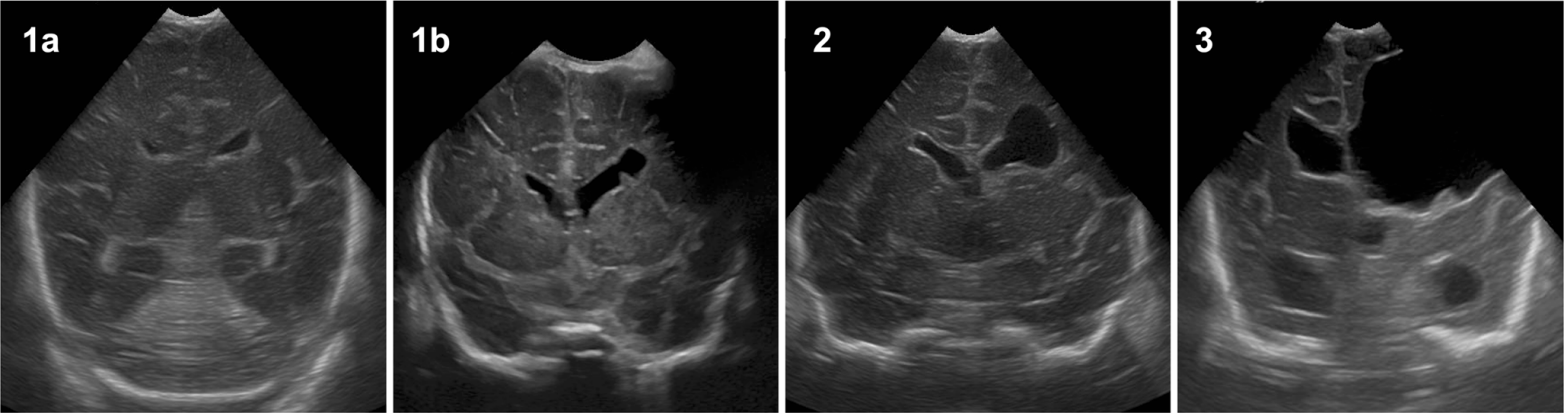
Classification of white matter damage by cystic lesion size at discharge: Exemplary coronal cerebral ultrasound slices of cystic white matter damage at time of discharge (postmenstrual age of 41 ± 5 weeks). 1a Gliosis optionally with cystic defects without communication to the ventricles. 1b Small cystic defect with communication to the ventricles. 2 Moderately sized cystic defect with communication to the ventricles. 3 Large cystic defect with communication to the ventricles
